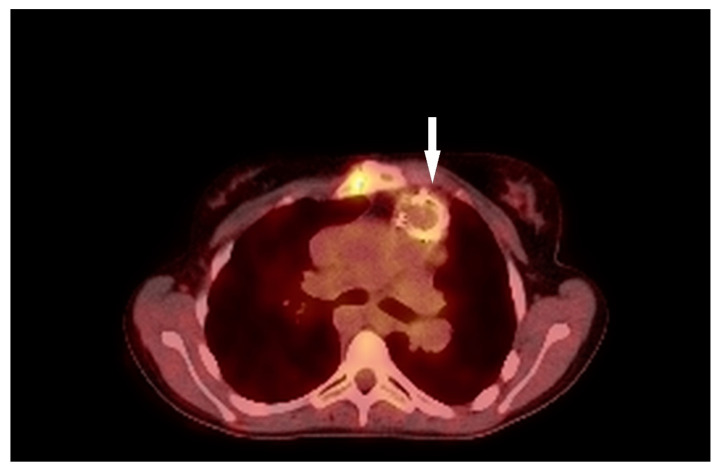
Positron emission tomography/computed tomography; The arrow point at Resilia 23 Edwards metal frame.
